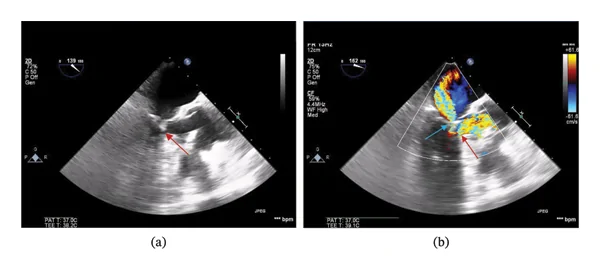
(a) Midesophageal long‐axis view in systole demonstrating SAM of the anterior mitral valve leaflet (red arrow). (b) Midesophageal long‐axis view in systole with color flow Doppler demonstrating flow acceleration through the left ventricular outflow tract (red arrow) and posteriorly directed mitral regurgitation jet secondary to SAM (blue arrow).
